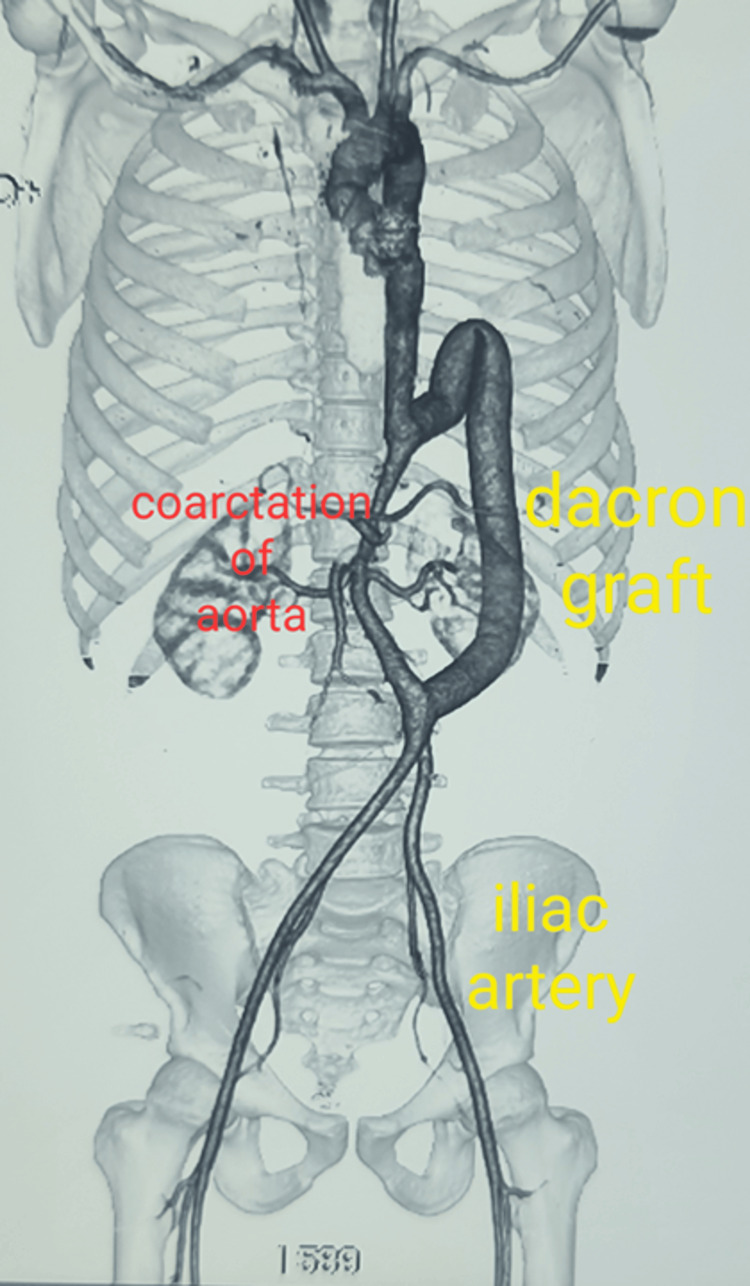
CT aortogram at the five-year follow-up showing good flow in the Dacron graft and good distal flow
